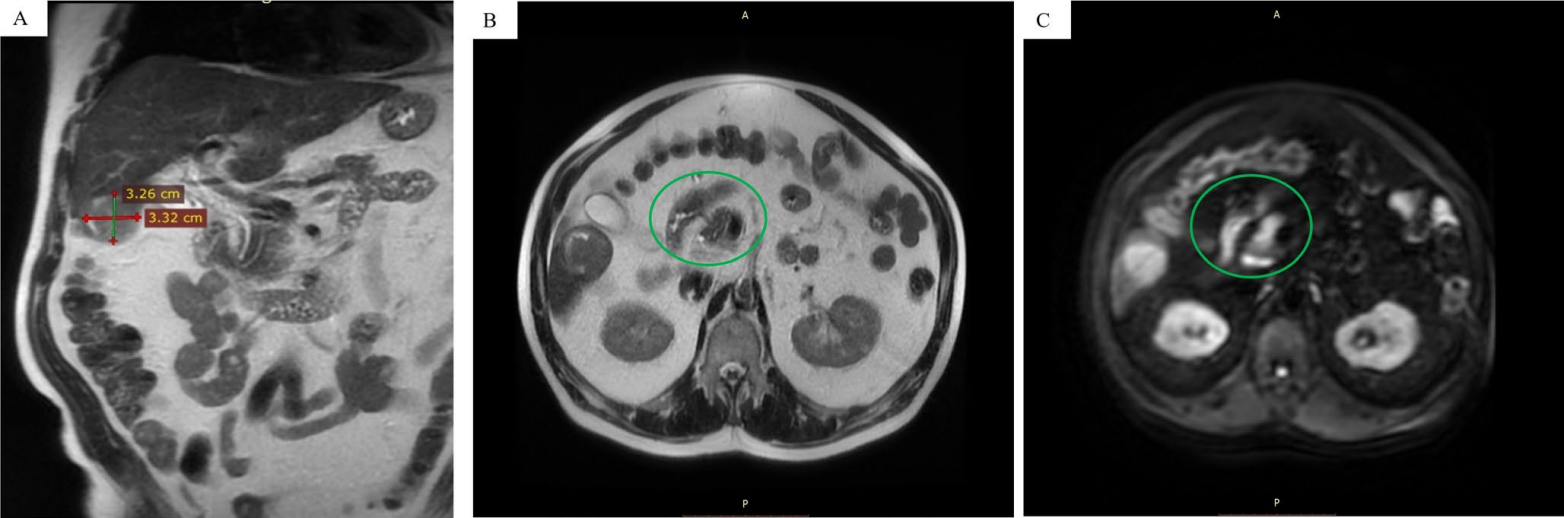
Abdominal magnetic resonance imaging (MRI) indicates a tumor mass located in the sixth segment of the liver—(A) Hyperintense round/oval nodule in the right lobe; (B) heterogeneity appearance of the HCC; and (C) DWI shows diffusion restriction of the nodule.
